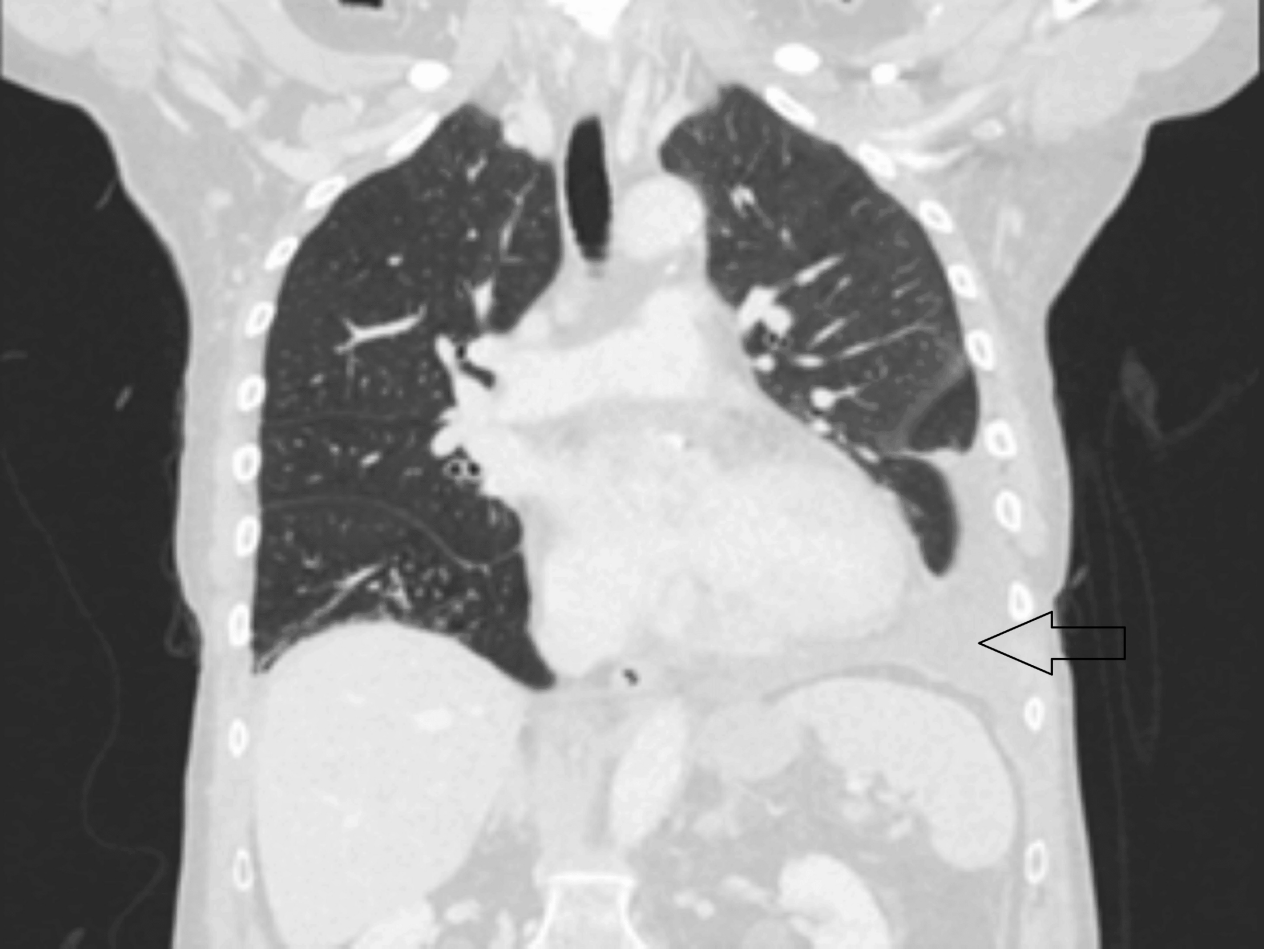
CT scan of the thorax and abdomen with contrast (coronal view) showing a moderate-sized left-sided pleural effusion with subtle pleural thickening.
